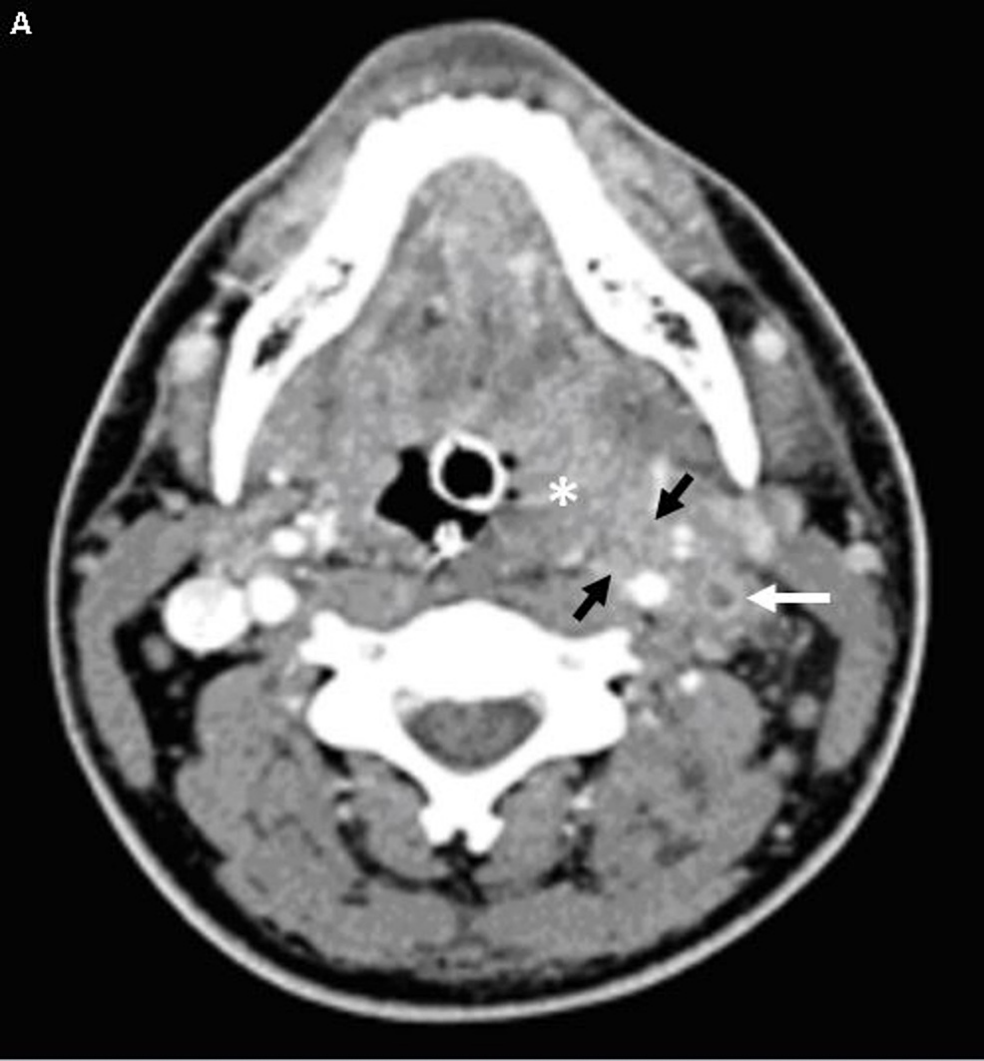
CT neck with IV contrast Axial contrast-enhanced CT of the neck shows an enlarged, hyperenhancing left palatine tonsil suggestive of acute tonsillitis (*). Inflammatory changes (black arrows) spread to the adjacent left carotid space with loss of the normal fat planes. Nonopacificiation of the left internal jugular with a hyperenhancing wall and surrounding soft tissue correspond to thrombosis and thrombophlebitis CT: computed tomography; IV: intravenous
